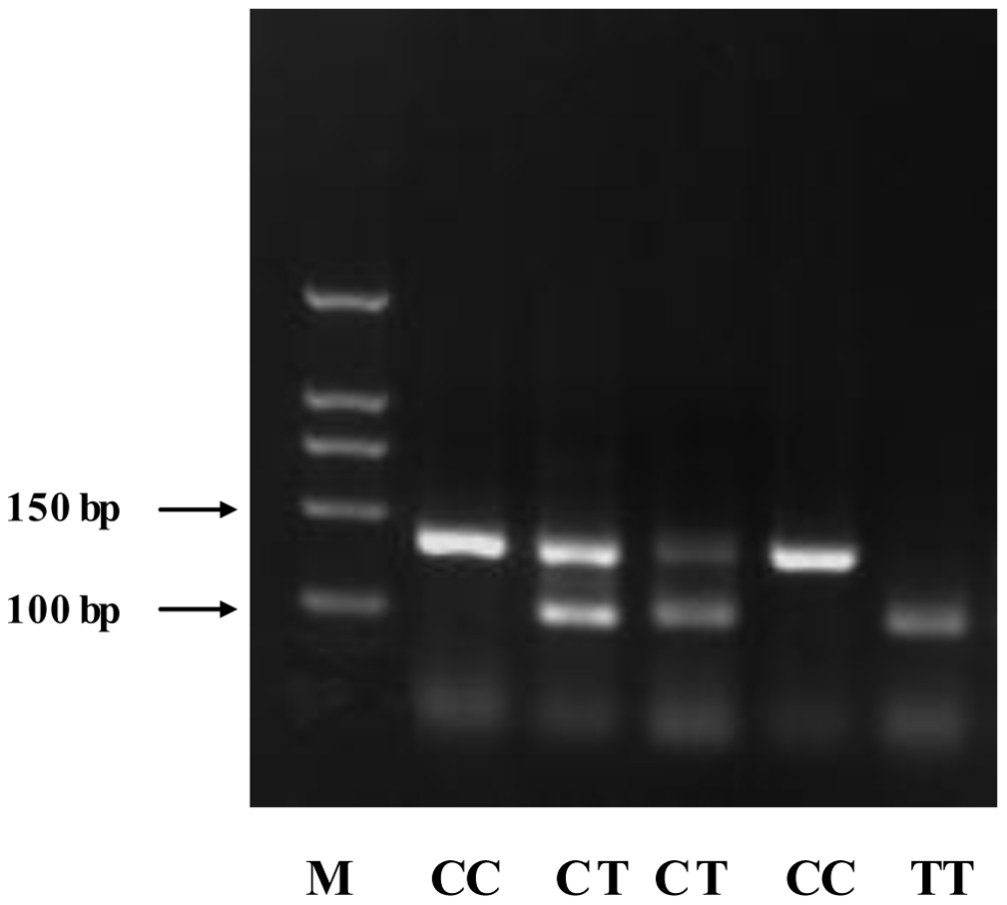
The image of genotyping result for rs7903146. Line 1: Maker; Line 2: CC genotype-139 bp; Line 3: CT genotype-139/111 bp; Line4: CT genotype-139/111 bp; Line 5: CC genotype-139 bp; Line 6: TT genotype-111 bp.
